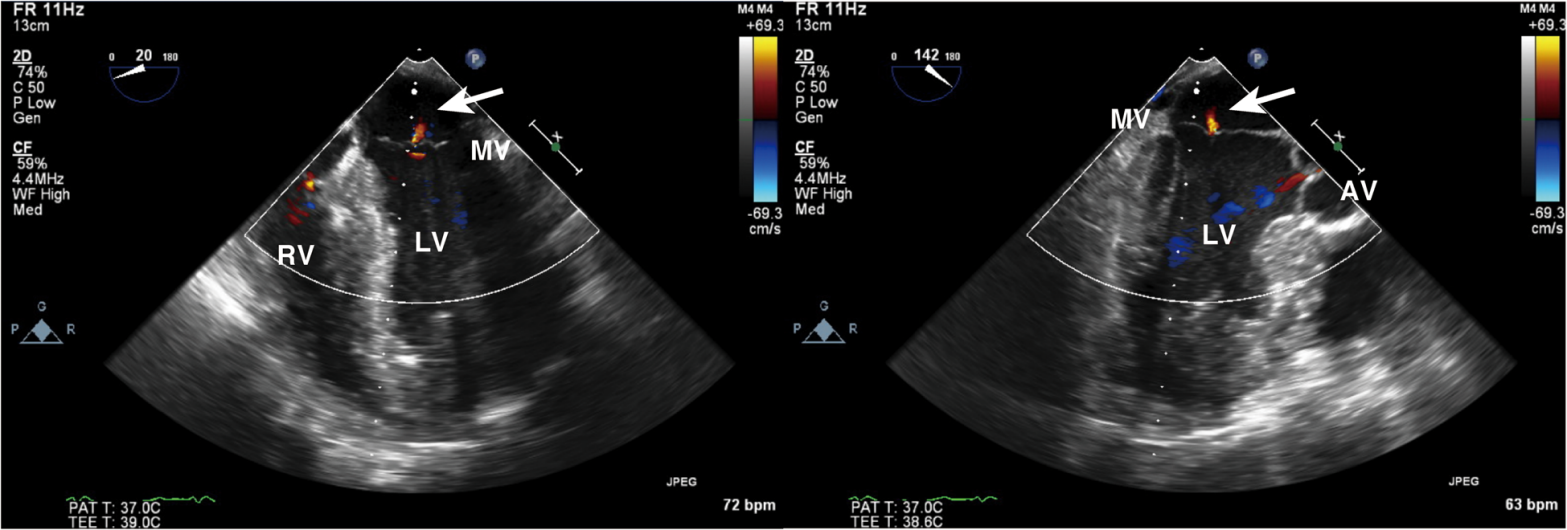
Color-flow Doppler images of mid-esophageal four chamber view (left) and midesophageal long axis view (right) after weaning from cardiopulmonary bypass. There is trivial mitral regurgitation (arrow) after the repair. AV aortic valve, LV left ventricle, MV mitral valve, RV right ventricle
